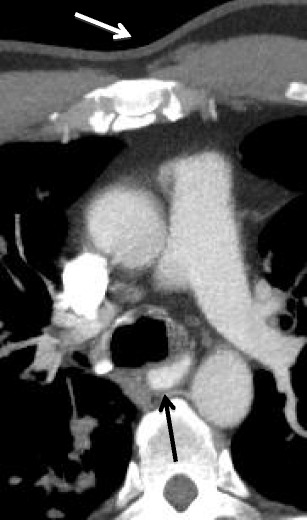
Axial CT demonstrating mucosal thickening with an enhancing lesion of the posterolateral trachea (*black arrow*) as well as overlying chest wall skin thickening (*white arrow*) in a patient with Kaposi sarcoma
